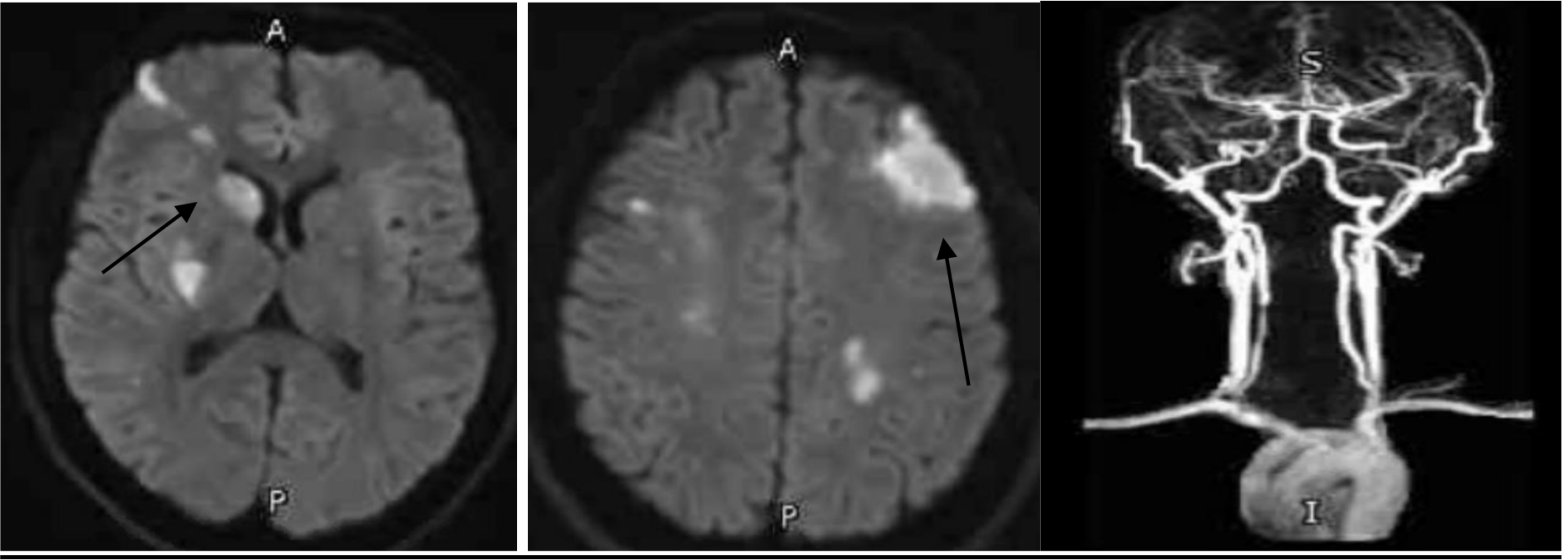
MRI, MRA head, and neck vessels showed multiple, variable-sized areas of acute ischemic strokes seen in the right frontal lobe, basal ganglia, corona radiata, and centrum semiovale along the MCA territory, and the similar regions are seen in the left frontoparietal lobes. MRA showed bilateral severe stenosis of the upper cervical ICA and cranial right ICA. The visualized parts of the right MCA and ACA are markedly attenuated. There is stenosis of the middle 1/3 of the cervical right vertebral artery.
